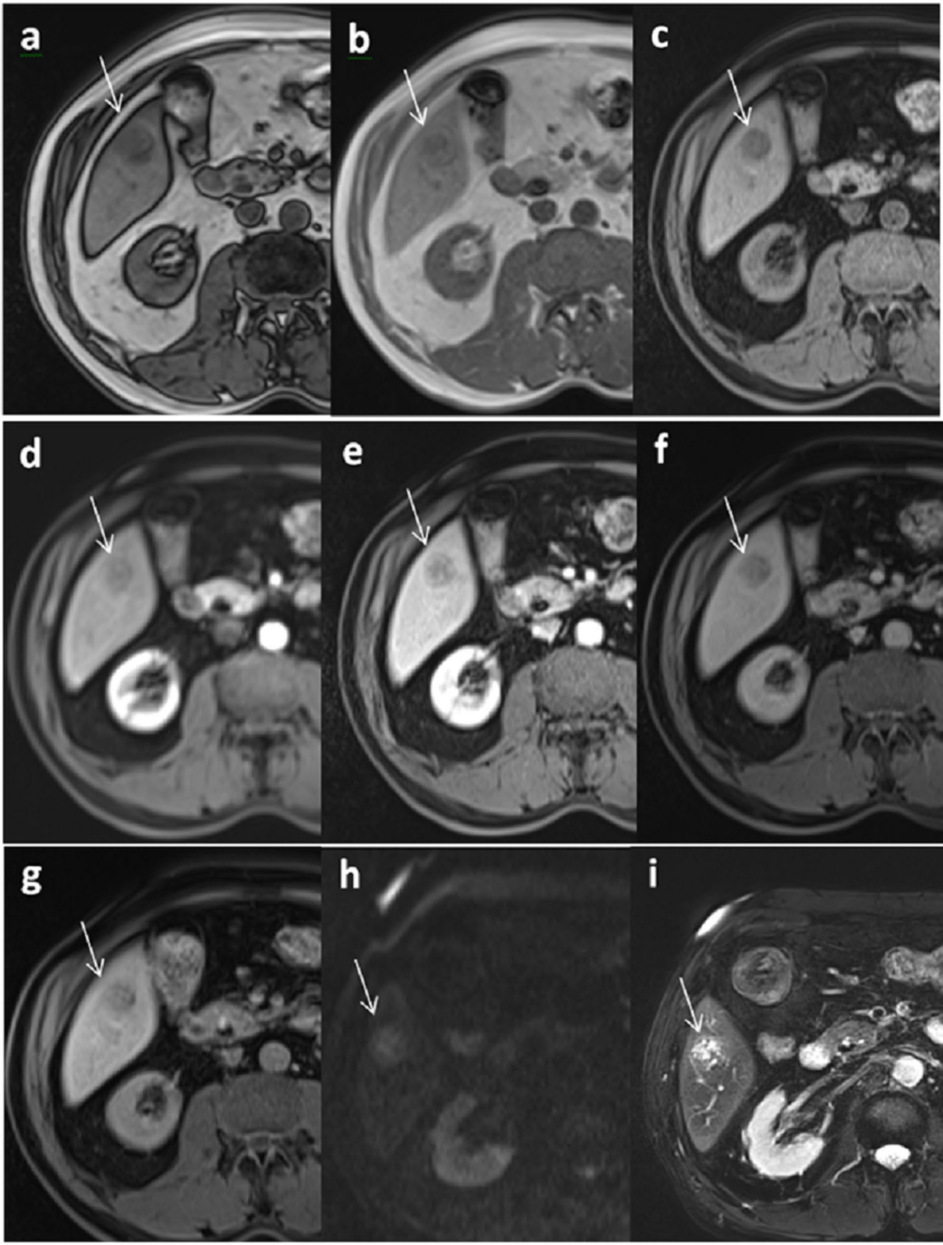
A male patient with chronic hepatitis B and a surgically confirmed hepatocellular carcinoma. a, b showed that the signal in the out-phase of the lesion reduced compared with that in the in-phase, indicating that the lesion contains fat. T1WI shows hypointensity (c), no obvious enhancement in the late arterial phase (AP) (d), and the enhancement degree in the portal venous phase (PVP) was still hypointensity (e), TP phase (f) and HBP phase (g) were hypointensity, and both T2WI (i) and DWI (h) showed hyperintensity. The nodule was 1.5 cm and was classified into LR-4 basing on the major features. Whenusing AF-HCC to classify the nodule, it can be upgraded, especially for the feature of TP hypointensity. This nodule was confirmed as hepatocellular carcinoma after surgical resection
